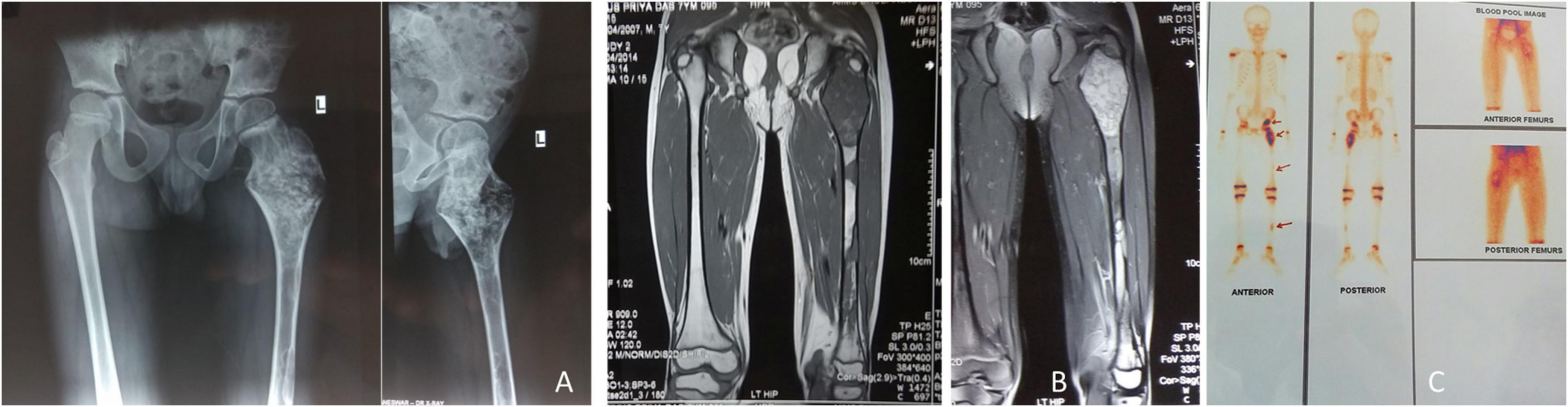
A 7-year-old male child presented with (PFD) involving left proximal femur, femoral shaft, left tibia and pelvis (A). MRI showed T2W hyperintense signal, and T1W hypointense signal (B). Three-phase bone scan revealed increased uptake at the involved sites (C).
